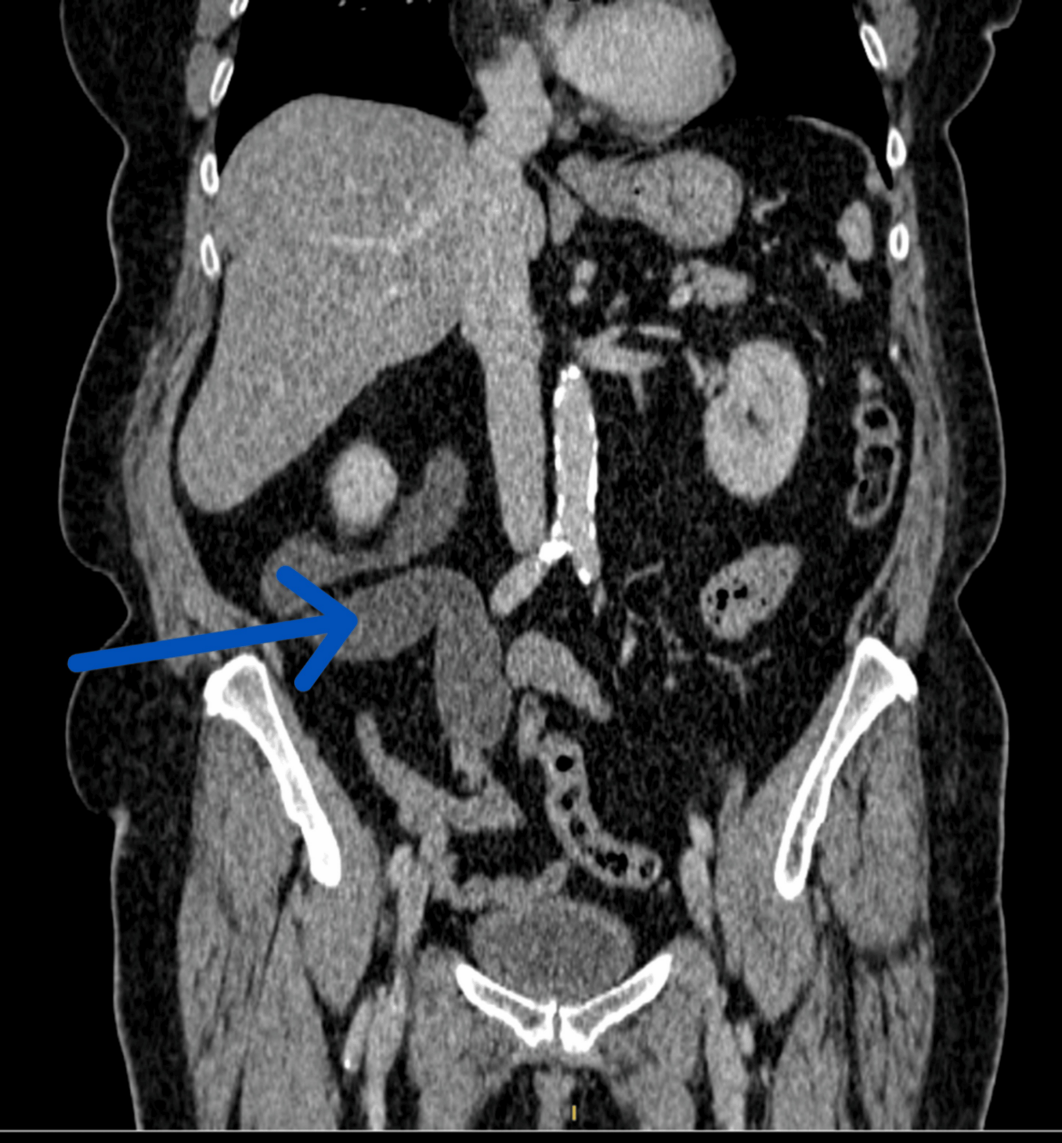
CT coronal imaging done eight years prior to attending the HOS (Haematuria One Stop) Urology Clinic The blue arrow shows the tortuous dilated right ureter.
